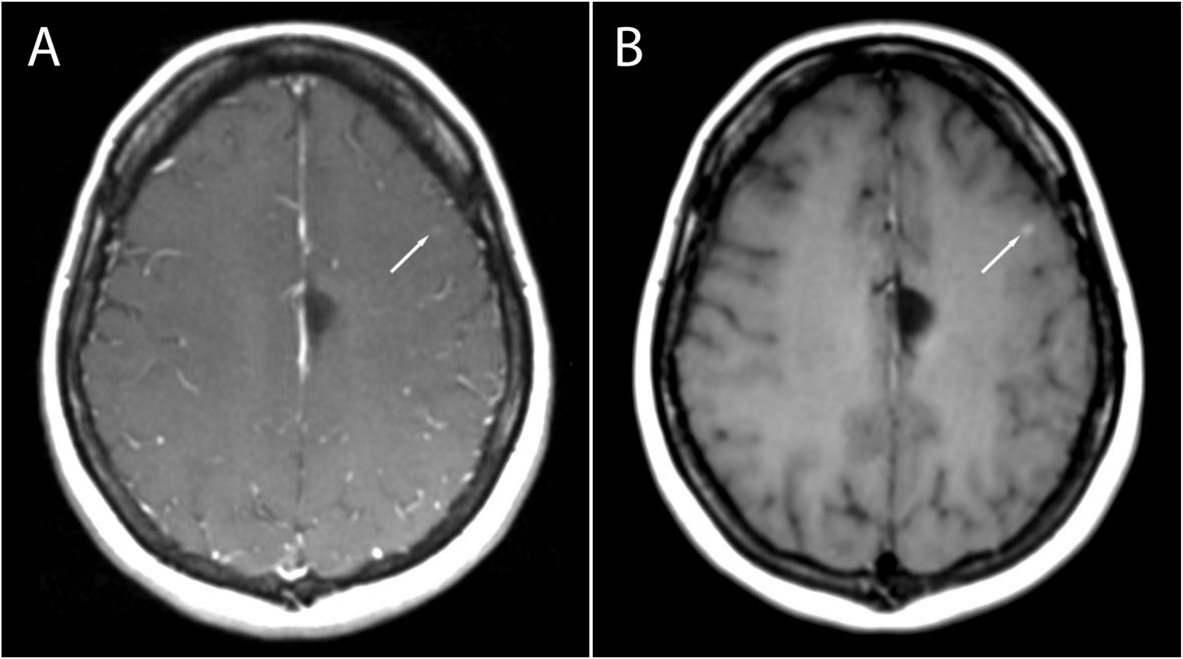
Small metastasis in the left frontal region in a 39-year-old female at 1.5 T, Gadovist 0.1 mL/kg. A T1-weighted SE sequence. B T1-weighted SPACE sequence. The SE sequence was acquired first
